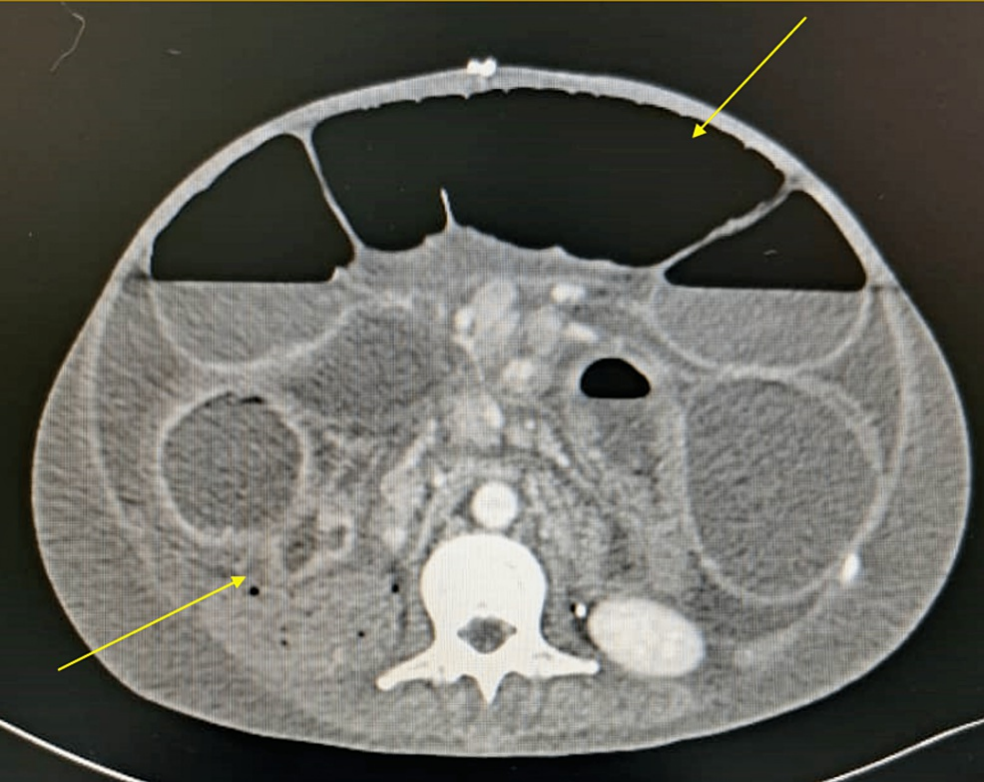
Abdominal CT scan with axial section showing a clear radiological occlusion with stenosis at 15 cm from the last ileal loop. CT: computed tomography
